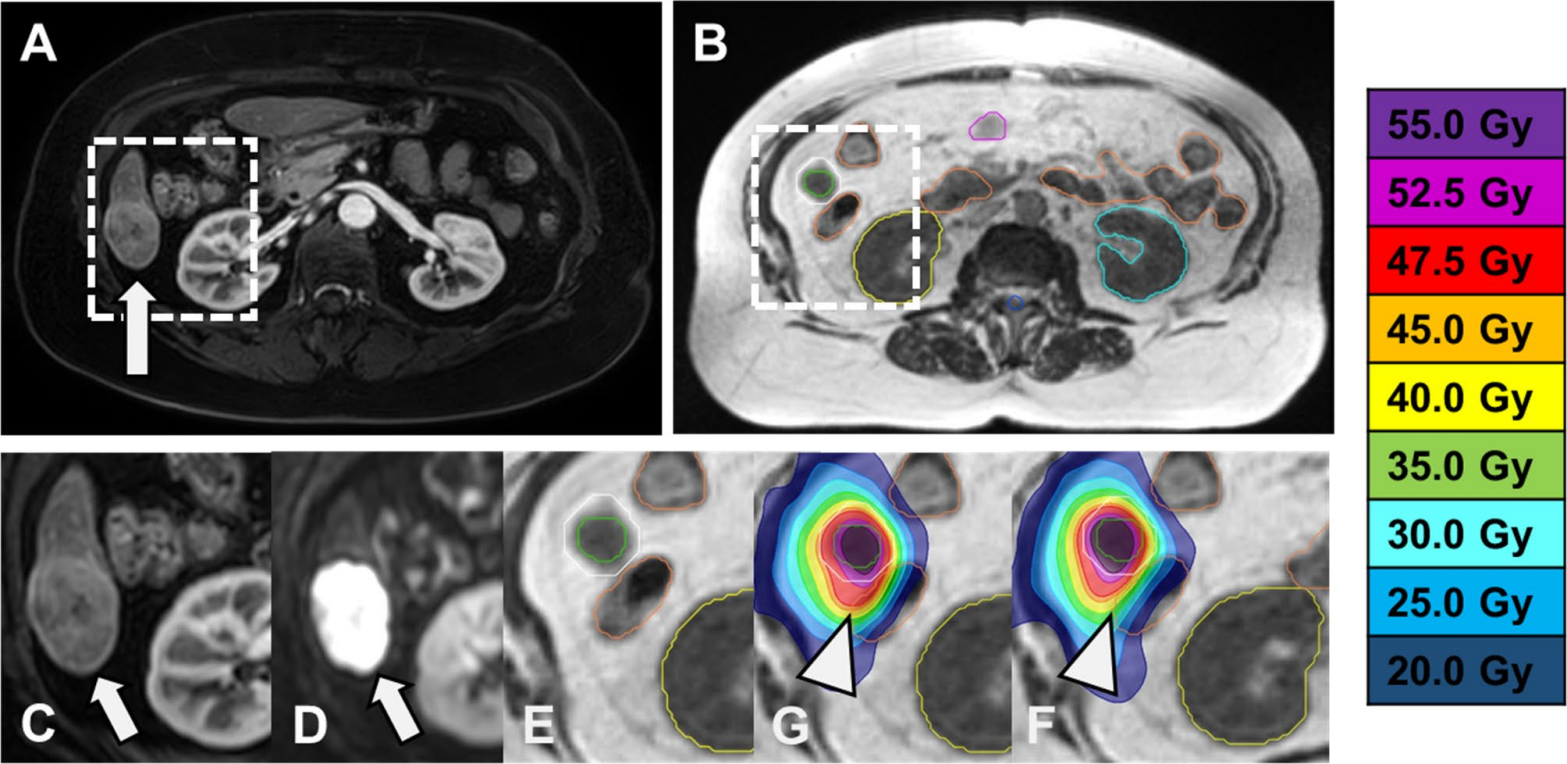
Case study: SMART of a liver metastasis. (A) Diagnostic T1w contrast enhanced (CE) MRI with liver metastasis in the caudal segment VI (white arrow). (B) Baseline imaging at the MR-Linac (TrueFISP). (C) – G) Zoom into the white frames in A) and B), with C) Diagnostic T1w CE MRI, (D) Diagnostic diffusion-weighted MRI (b = 600 s/mm²), (E) MR-Linac TrueFISP, (F) deformable image registration (DIR)-based dose accumulation of predicted plans (theoretical non-adaptive treatment) with overdose inside the intestines (white arrowhead) and (G) DIR-based dose accumulation of adapted plans with protection of the intestines (white arrowhead)
